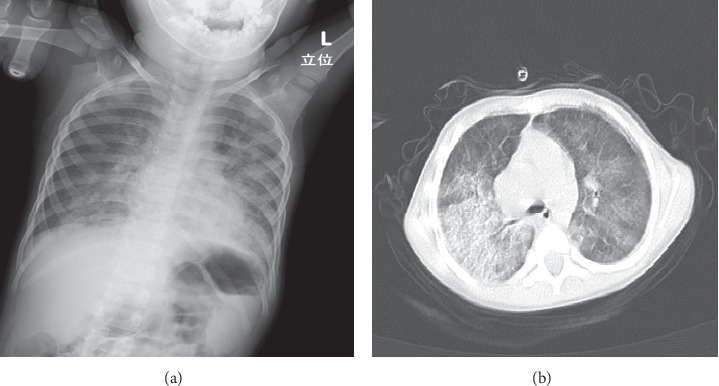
(a) Posteroanterior chest radiography demonstrating bilateral pulmonary infiltrations at three months after first acute alveolar hemorrhage. (b) Axial CT scan of the thorax showing diffuse ground-glass appearance.
